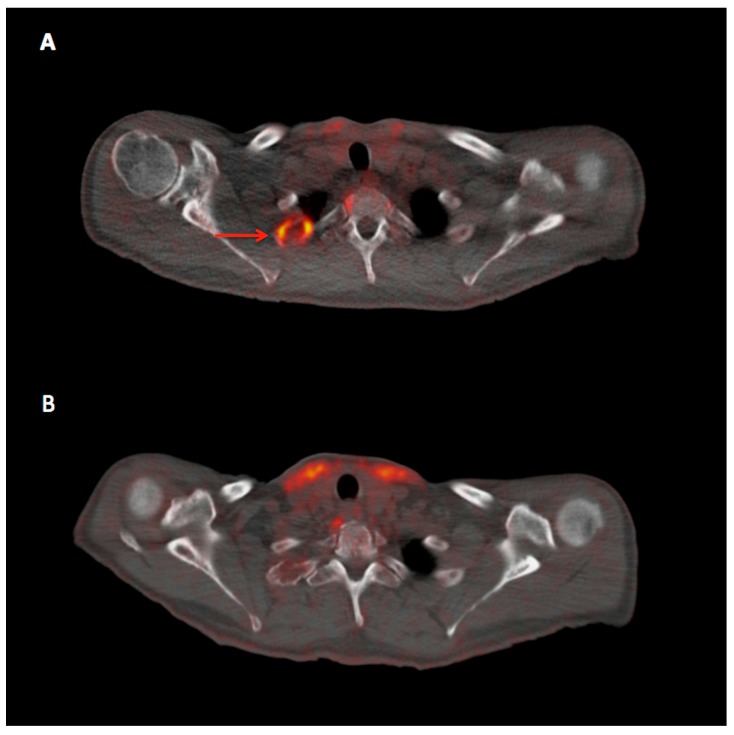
Transaxial fused ^18^F-fluoro-2-deoxy-d-glucose positron emission tomography/computed tomography (^18^F-FDG PET/CT) images of the relapsed 73-year-old multiple myeloma (MM) patient before (A) and after (B) three cycles of combination treatment with elotuzumab, lenalidomide, and dexamethasone. The patient demonstrated, among others, a highly ^18^F-FDG avid, osteolytic lesion in the second rib on the right before initiation of the new treatment (arrow, A), which responded with an almost complete metabolic remission after three cycles (B). The previously thrice treated with high-dose chemotherapy and autologous stem cell transplantation patient showed a major decrease of the serological myeloma activity parameters as response to the combined treatment: serum monoclonal protein decreased from 4.6 g/L to detectable but not quantifiable levels, kappa light chains decreased from 37.6 mg/L to 22.7 mg/L, and the free light chains (FLC) ratio was normalized from 3.2 to 1.6. Thus, the imaging findings on follow-up PET/CT were considered in line with the serological response. Elotuzumab is a humanized immunostimulatory antibody that recognizes lymphocytic activation molecule F7 (SLAMF7), a surface glycoprotein expressed by myeloma and natural killer cells, and elicits a dual effect by directly activating natural killer cells and mediating antibody-dependent cell-mediated cytotoxicity via the CD16 pathway to cause targeted myeloma cell death [1]. Elotuzumab is the first monoclonal antibody approved for the treatment of relapsed-refractory MM in combination with lenalidomide, an immunodulatory drug, and dexamethasone. The combination of elotuzumab, lenalidomide, and dexamethasone leads to an improved progression-free survival, overall response rate and interim overall survival in patients with relapsed or refractory MM, as compared to the standard regiment of lenalidomide and dexamethasone [2,3].
